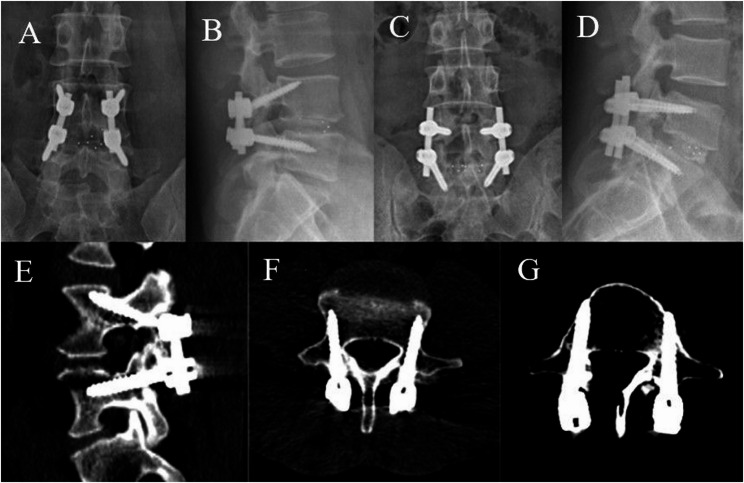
Anteroposterior and lateral radiographs after MCBT screw and TASS fixation (A, B) and pedicle screw fixation (C, D). E and F, Axial and sagittal computed tomography images of the L4 cortical bone trajectory. E and G, Axial and sagittal computed tomography images of the L5 TASS trajectory
